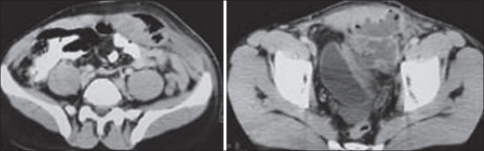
CT scan shows loculation with air pockets in the left lower rectus sheath and displacement of urinary bladder
